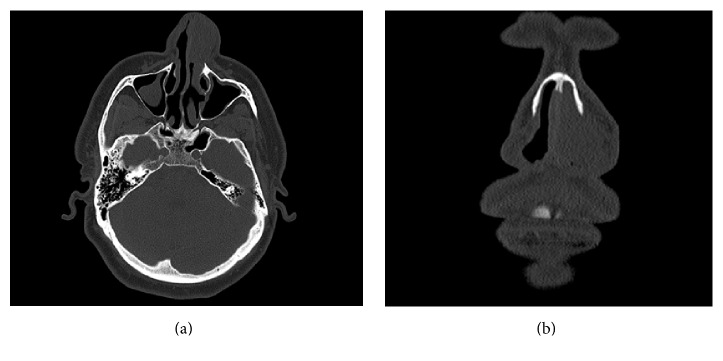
(a) shows axial and (b) shows coronal CT images. Both show a soft tissue mass occupying the left nasal vestibular area.
